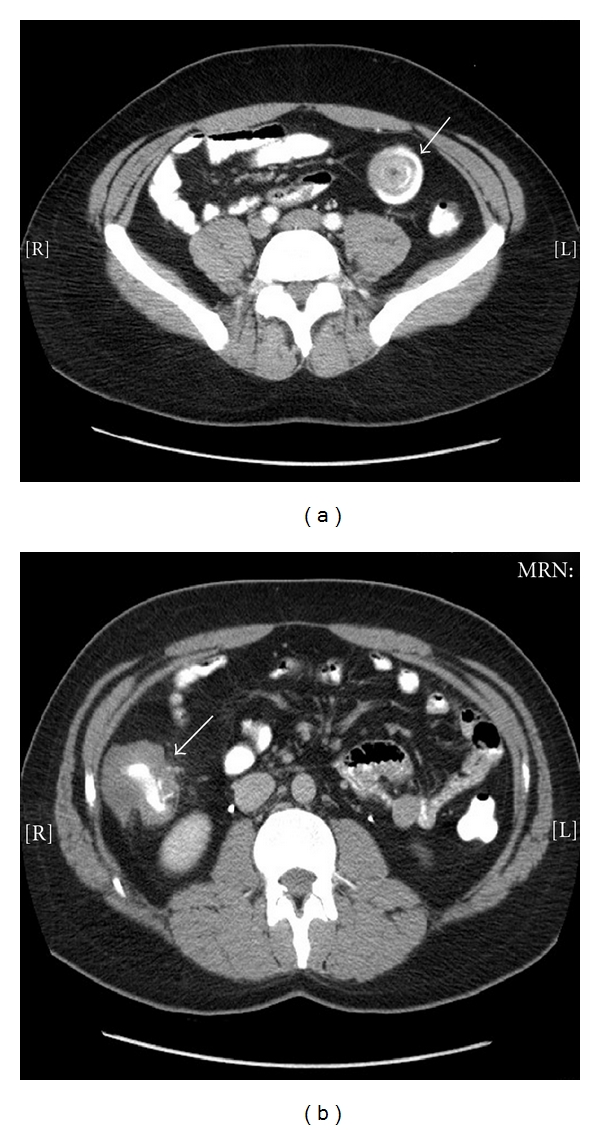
CT scan revealed intussusception of the small bowel (a) and thickening of the right colon (b).
